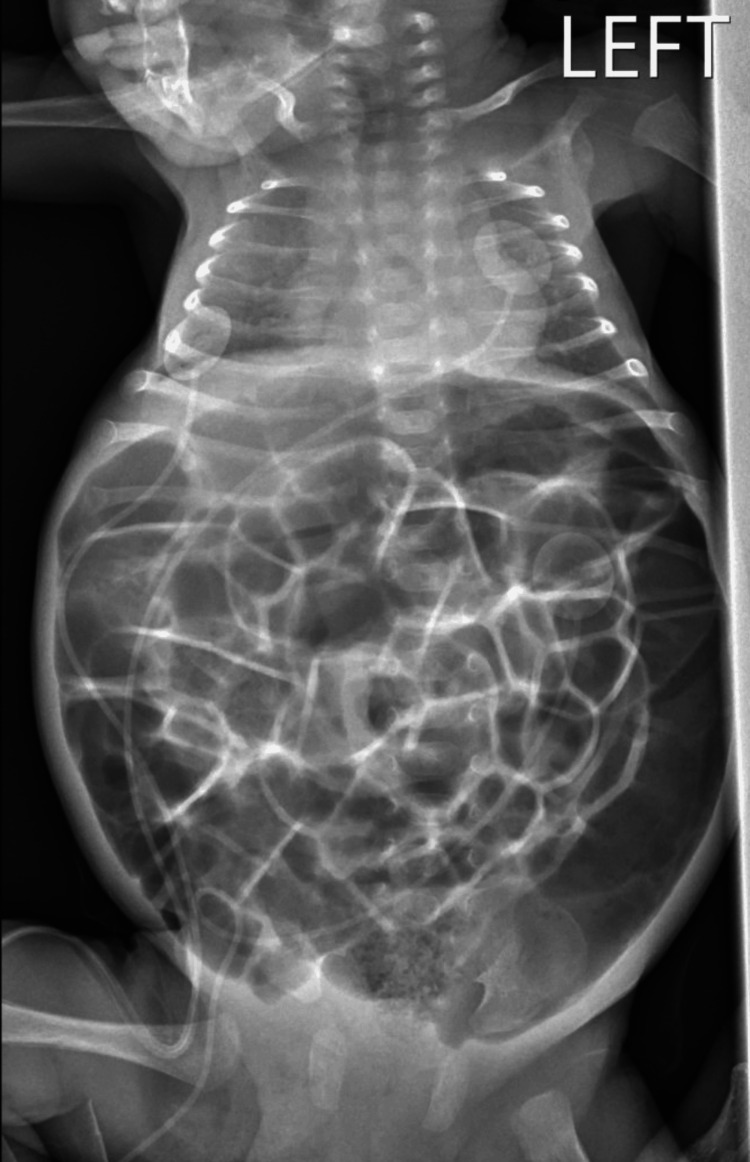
Supine anteroposterior radiograph of chest and abdomen demonstrating moderate gaseous distension suggestive of generalized ileus
